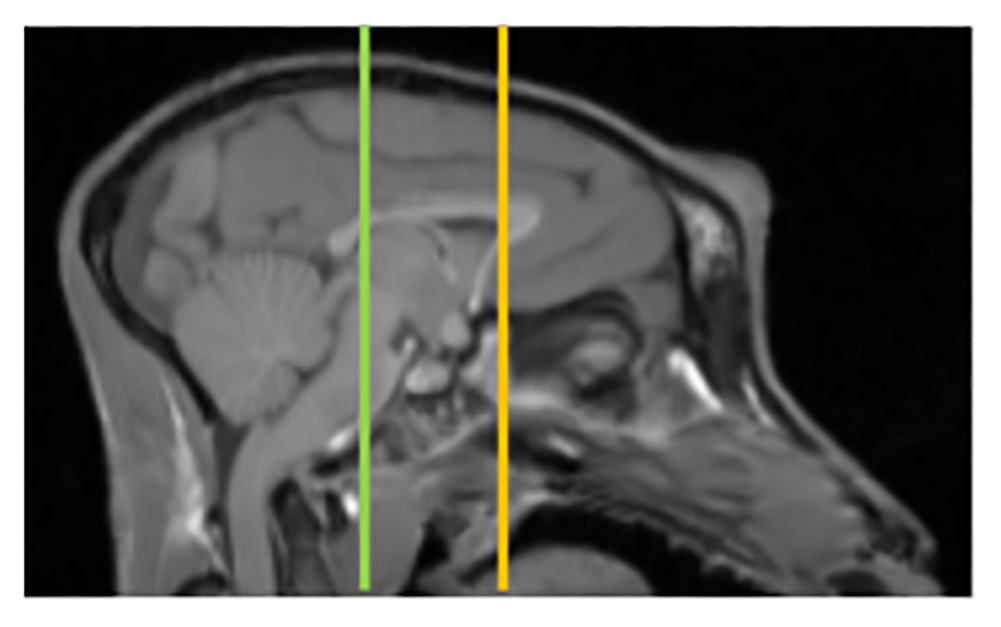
An example of how anterior cingulate cortex and posterior cingulate cortex were distinguished for tracing. A sagittal slice of a T1 weighted MR image is shown with vertical lines for the posterior commissure (green vertical line) and the most anterior part of the genu of the corpus callosum (yellow vertical line). The coronal slices used for the anterior cingulate cortex (ACC) were the five centered around the coronal slice containing the most anterior part of the genu of the corpus callosum. The coronal slices used for the posterior cingulate cortex (PCC) were the five centered around the coronal slice containing the posterior commissure.
